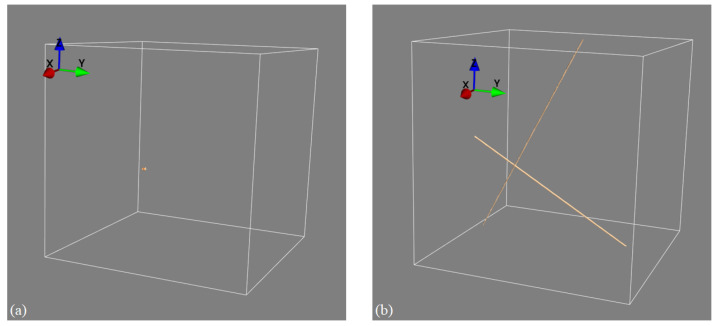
Differences between stereo X-ray tomography and limited-view tomography. (a) Stereo XCT reconstruction of a point-like object. (b) FBP reconstruction of the same object; the object’s information is smeared out along the X-ray paths that intersect the object.
